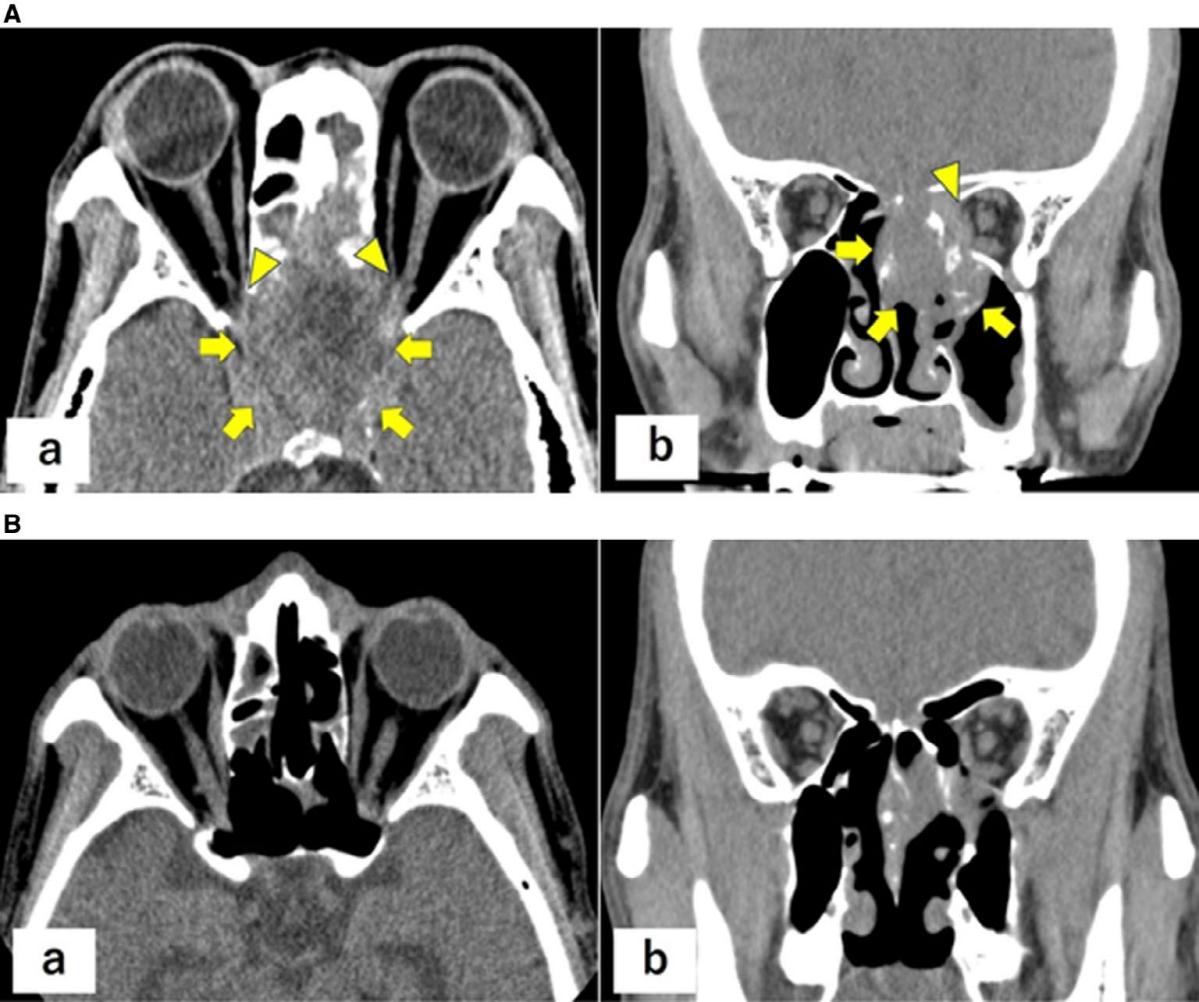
FIGURE 3 (A) Non‐contrast head computed tomography images showing interval enlargement of the sphenoid sinus tumor with involvement of both optic canals, consistent with optic pathway compromise. (a) Axial image. (b) Coronal image. Arrows: tumor; arrowheads: optic canals. (B) Non‐contrast head computed tomography images during follow‐up demonstrating interval shrinkage of the sphenoid sinus lesion after radiotherapy and immunotherapy, consistent with a partial response. (a) Axial image. (b) Coronal image.
